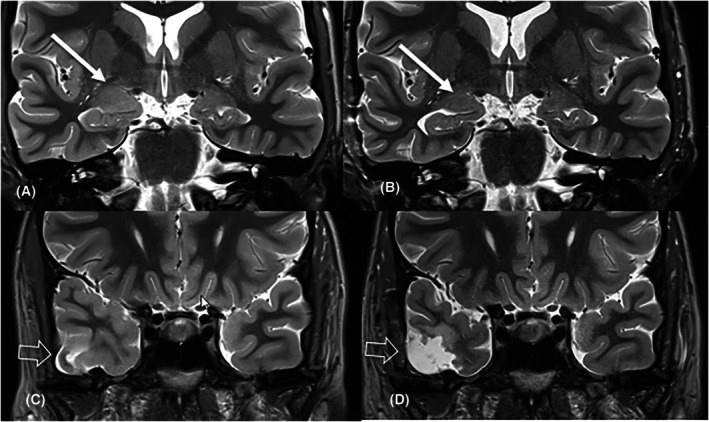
A 22 year‐old woman (#34) presented with focal seizures with preserved and impaired consciousness. MRI showed right‐sided amygdala enlargement and a ganglioglioma of the inferior temporal gyrus. Six months after resection she was seizure‐free and amygdala enlargement had regressed.
